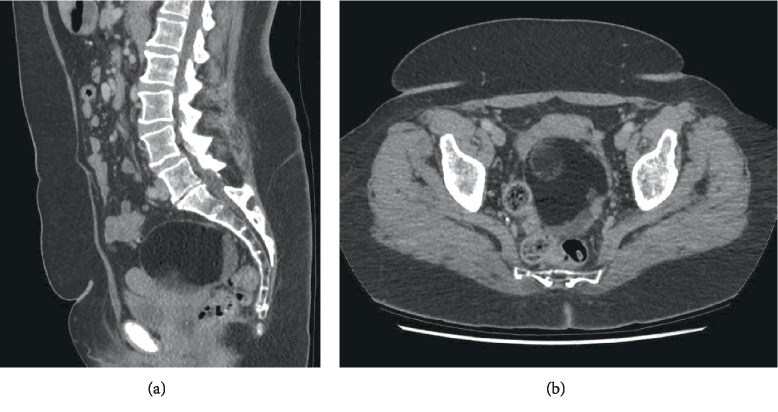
Preoperative CT images of the right adnexal mass.
